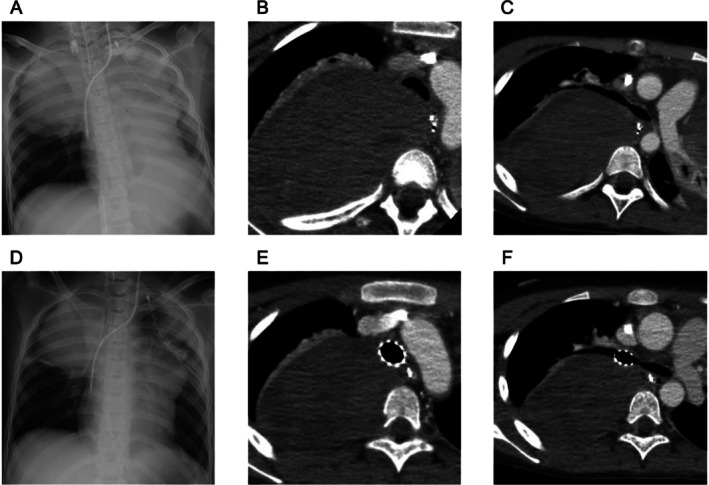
Chest radiograph reveals a large right upper lung zone mass measuring 83 mm × 75 mm with extensive bilateral atelectasis (A). Computed tomography (CT) imaging demonstrates compression and stenosis of the trachea and right main bronchus by the tumour (B, C). Two metallic stents were implanted in the trachea and right main bronchus (D–F).
